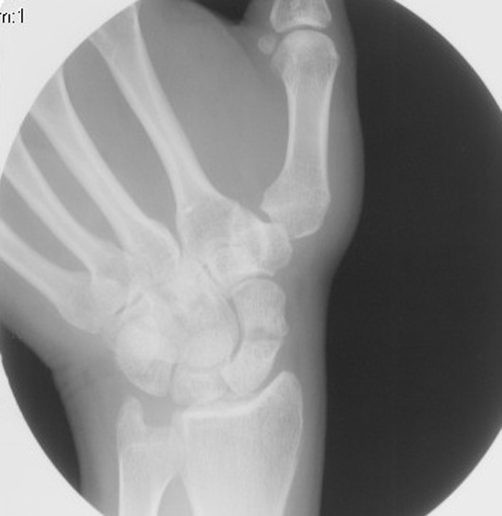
Radiograph of Case #1. Transverse fracture through waist of scaphoid occurring one year previously. No displacement or avascular necrosis is evident.
